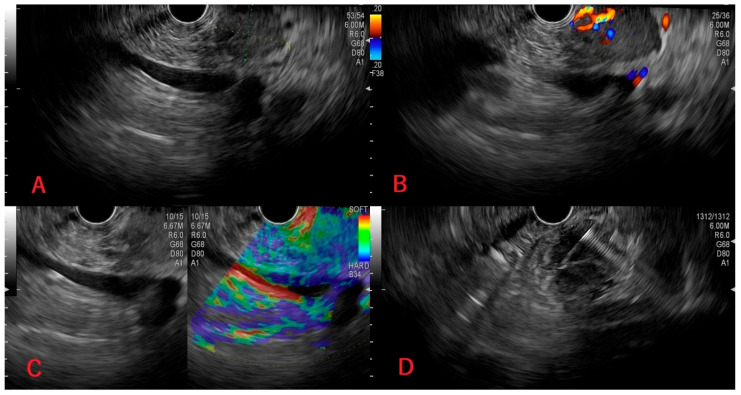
Case 1: Hypoechoic pancreatic tumor measuring 21/16 mm (A), intense Doppler signal (B), hard on elastography (C); tissue acquisition through EUS-FNB (D).
